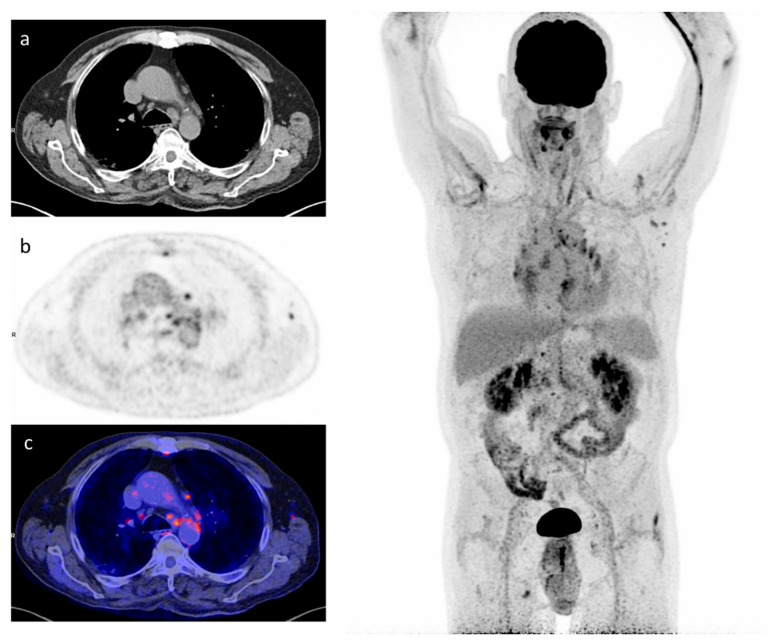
A 64-year man admitted for multilobar pneumonia caused by SARS-CoV-2. The [^18^F]FDG-PET/CT at 3 months after symptom onset shows increased [^18^F]FDG uptake in residual pulmonary lesions (TLG 124,11) and mediastinum lymph node (SUVpeak 1,73). Pulmonary function tests evidenced severe pulmonary diffusion impairment, with a diffusing capacity of the lungs for carbon monoxide 41% of the predicted value. Left: (a) CT transverse slice, (b) [^18^F]FDG-PET slice, and (c) fused [^18^F]FDG-PET and CT images. Right: whole-body, maximal intensity projection image, displaying mediastinal lymph nodes with [^18^F]FDG uptake.
